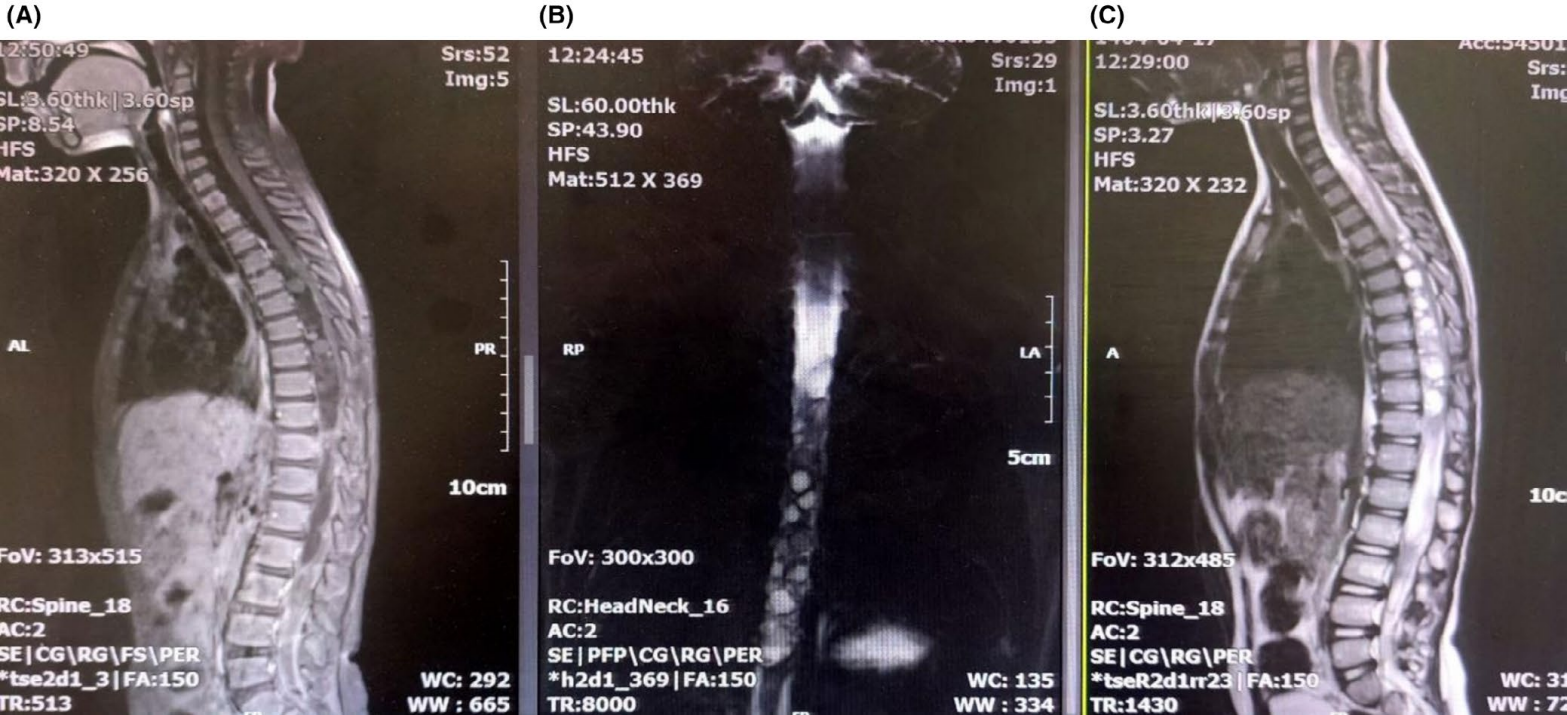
Postoperative thoracolumbar imaging after 10 months. (A) T1‐weighted MRI with gadolinium injection (left), (B) Myelogram (middle), (C) T2‐weighted MRI with gadolinium injection (right). Figures show recurrence of intradural multiple hydatid cysts with expansion to upper segments of thoracic spine.
